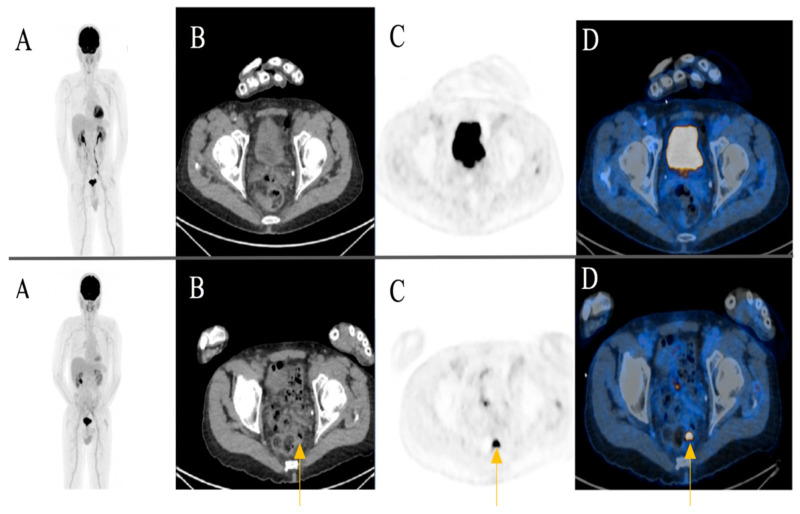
A 72-year-old male patient initially with metastatic cutaneous melanoma stage IIIC (T0 N2b M0). (A) Maximum intensity projection (MIP); (B) Computed Tomography (CT) axial of pelvis; (C) Positron Emission Tomography (PET) axial of pelvis; (D) Fused images PET/CT axial of pelvis. First row: FDG-PET/CT performed for extended staging after left axillary lymphadenectomy without evidence of metabolically active melanoma, nodal or distant metastasis. Second row: Follow-up for treatment response assessment under anti-PD1 monotherapy. Three months after treatment initiation, a new metabolically active wall thickening of the rectosigmoidal colon was seen (yellow arrow). A rectoscopy was subsequently recommended for further evaluation. The biopsy revealed a well-differentiated adenocarcinoma of the colon.
